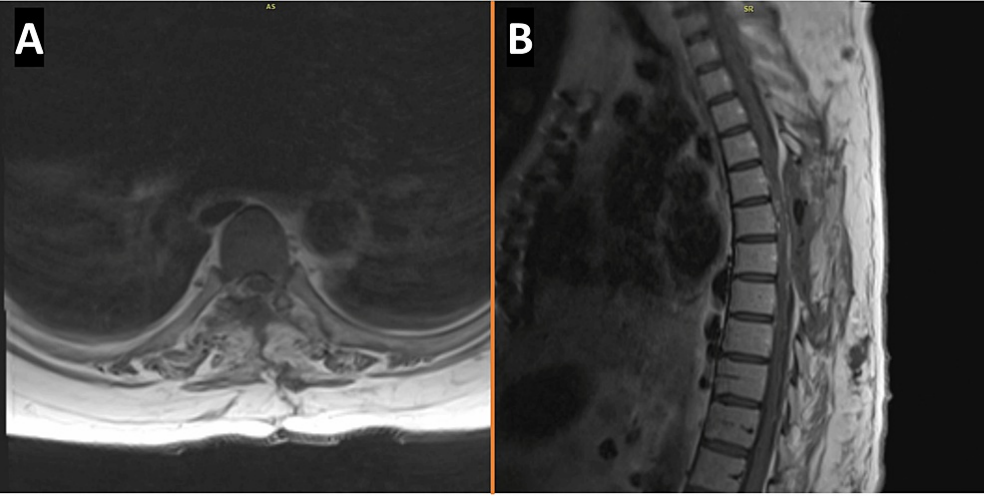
Postoperative T1-weighted post-contrast MRI in axial (A) and sagittal (B) views without residual tumor.
